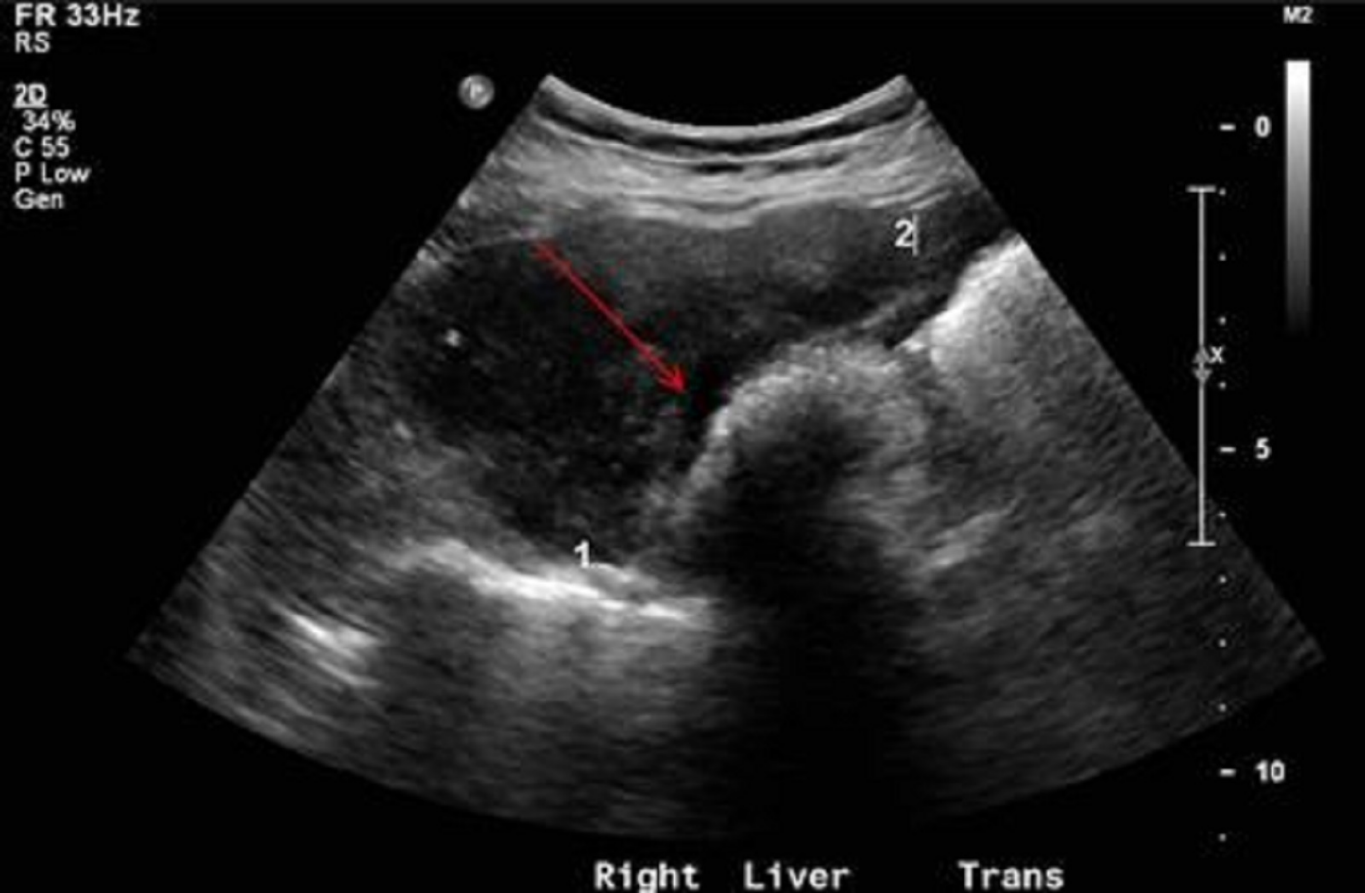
Companion case. Right upper quadrant ultrasound of a patient who presented a few months after the first patient. The image demonstrates a hypoechoic lesion near the expected location of the gallbladder (red arrow). Given the suspicious ultrasound finding and recent prior GBC case, leading differential diagnosis was neoplasm. As a result, further evaluation with a CT scan was recommended.
